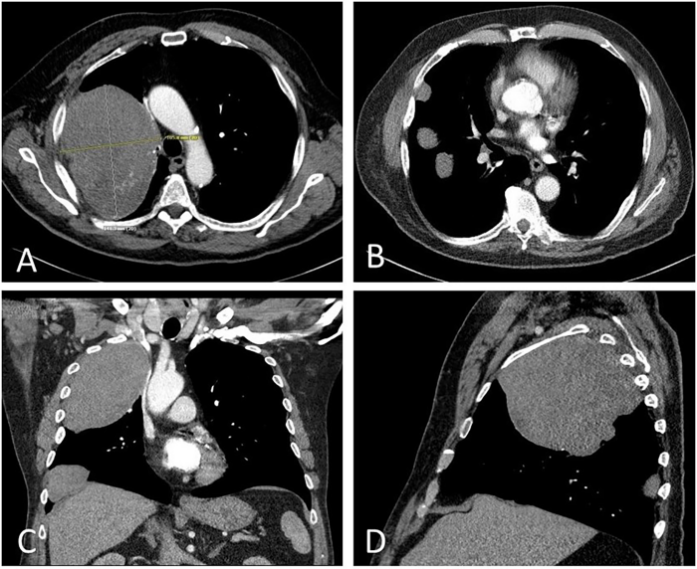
Computed tomography with axial (A and B), coronal (C) and sagittal (D) images showing 4 solid right pleural masses, the largest measuring 14 cm × 10 cm × 12 cm with heterogenous enhancement.
